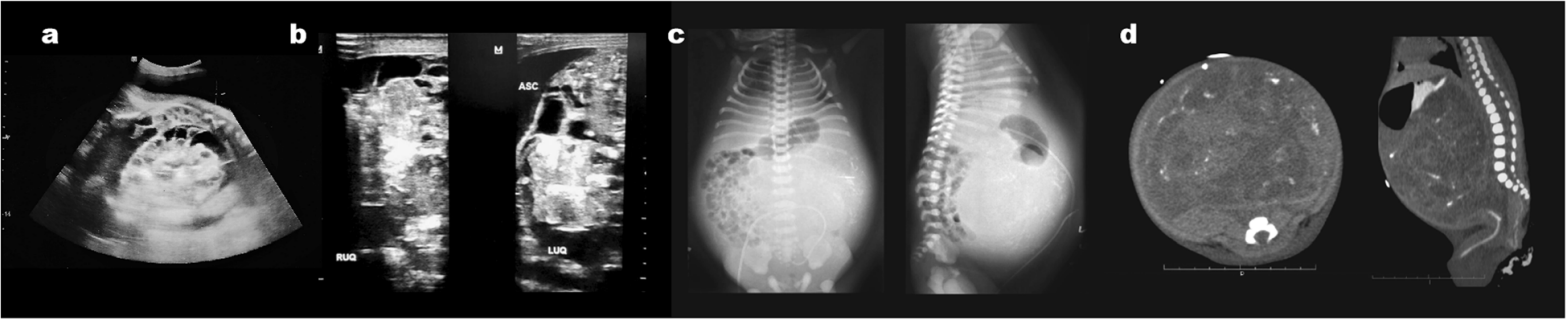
Imaging studies: a. Prenatal ultrasound showing fetal ascites and matted bowels with scattered calcifications; b. X-ray AP and lateral views indicating an intra-abdominal soft tissue density with calcifications, elevating the diaphragms and displacing the bowels to the right and posteriorly; c. Ultrasound images showing a large, complex abdominopelvic focus (9.4 cm in its widest dimension) with calcifications and moderate ascites (in the perihepatic, perisplenic and pelvic areas); d. CT transverse and sagittal views showing an intra-abdominal complex focus with internal septations and calcifications (measuring 10.1 × 13.2 × 8.2 cm), displaced viscerae, and moderate ascites
